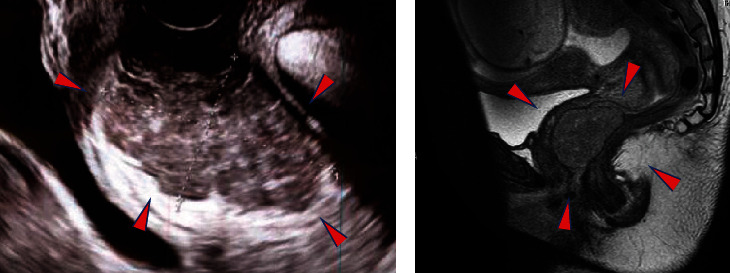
Radiographic images showing the mass. (a) Transvaginal ultrasound: the mass (arrowheads) was 51.7 × 32.5 mm in size. (b) Plain magnetic resonance image of the abdomen and pelvis. An irregular mass (arrowheads) can be seen superior to the vagina.
